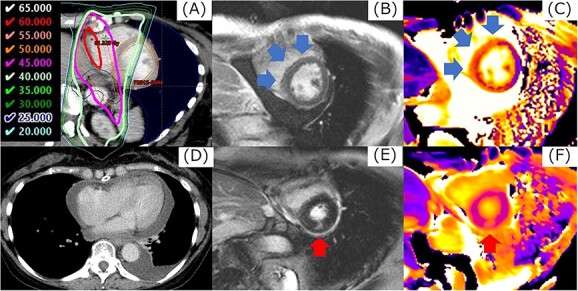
The example in cardiac event of Grade 4. Corresponding regions of 40 Gy or more (A), LGE by magnetic resonance imaging were detected in the mid-myocardial layer in the base of the LV (B). The value of native T1 in this region increased from 1168 ms before CRT to 1269 ms (C). At 53.7 months after CRT, the patient had pericardial effusion with cardiac tamponade (D). When CMR imaging was performed at the diagnosis of cardiac tamponade, a new LGE at a margin of dose distribution of 45 Gy was observed in the mid-myocardial layer in the base of the LV, separately from the LGE identified 6 months after CRT (E, F). Cardiac catheterization revealed constrictive pericarditis, and a pericardial incision was made.
